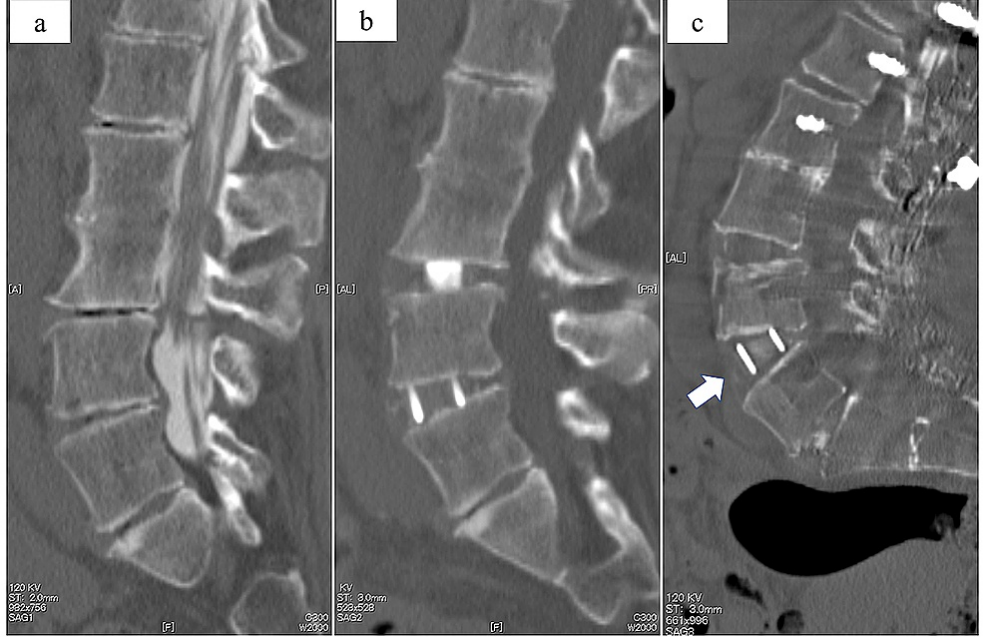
The segmental lordosis at L4/5 Computed tomography of the lumbar spine, sagittal plane preoperative imaging. The segmental lordotic angle at L4/5 was 9.7° preoperatively (a). After anterior fixation (XLIF) (b), the angle was 14°. After posterior fixation (c), the angle was 40° postoperatively with rupture of the anterior longitudinal ligament (white arrow).
